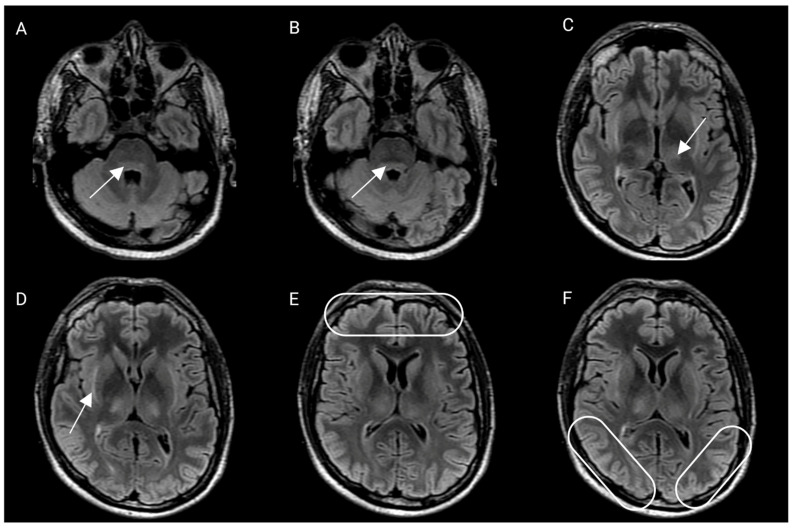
Brain magnetic resonance imaging, axial fluid-attenuated recovery sequence (FLAIR). Arrows and ovals show the areas described in each section. (A,B) There is a hyperintensity that shows a compromise of the dorsal pons at the level of the fourth ventricle, involving the nucleus of the VI and VII cranial nerves bilaterally. (C) There is a compromise of the thalamus at its ventromedial aspect, bilaterally. (D) Compromise of the subinsular external capsules. (E,F) There is evident cortical edema, most probably of cytotoxic origin.
